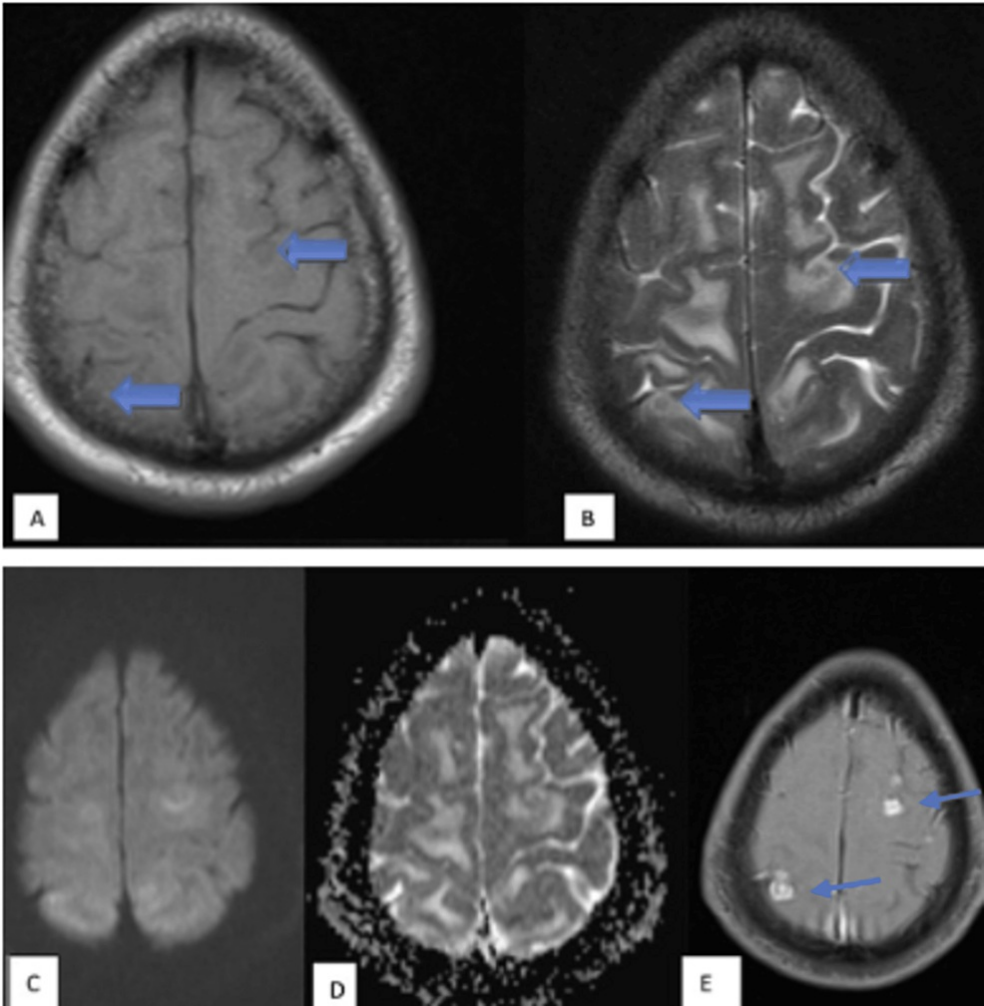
Tuberculoma - caseating granulomas without central liquefaction MRI images: (A) Axial T1WI, (B) axial T2WI, (C) axial DWI, (D) axial ADC map, and (E) axial T1-weighted post-contrast. These images show few, variable-sized, T1 iso and T2 hypointense lesions with T2 hypointense rim (blue arrows) and moderate perilesional edema in bilateral high parietal lobes with no restricted diffusion on DWI. The ADC value obtained was 1.1 x 10^-3^ mm^2^/s. On post-contrast, the lesions show thick ring enhancement. T1WI: T1-weighted imaging; T2WI: T2-weighted imaging; DWI: diffusion-weighted imaging; ADC: apparent diffusion coefficient.
